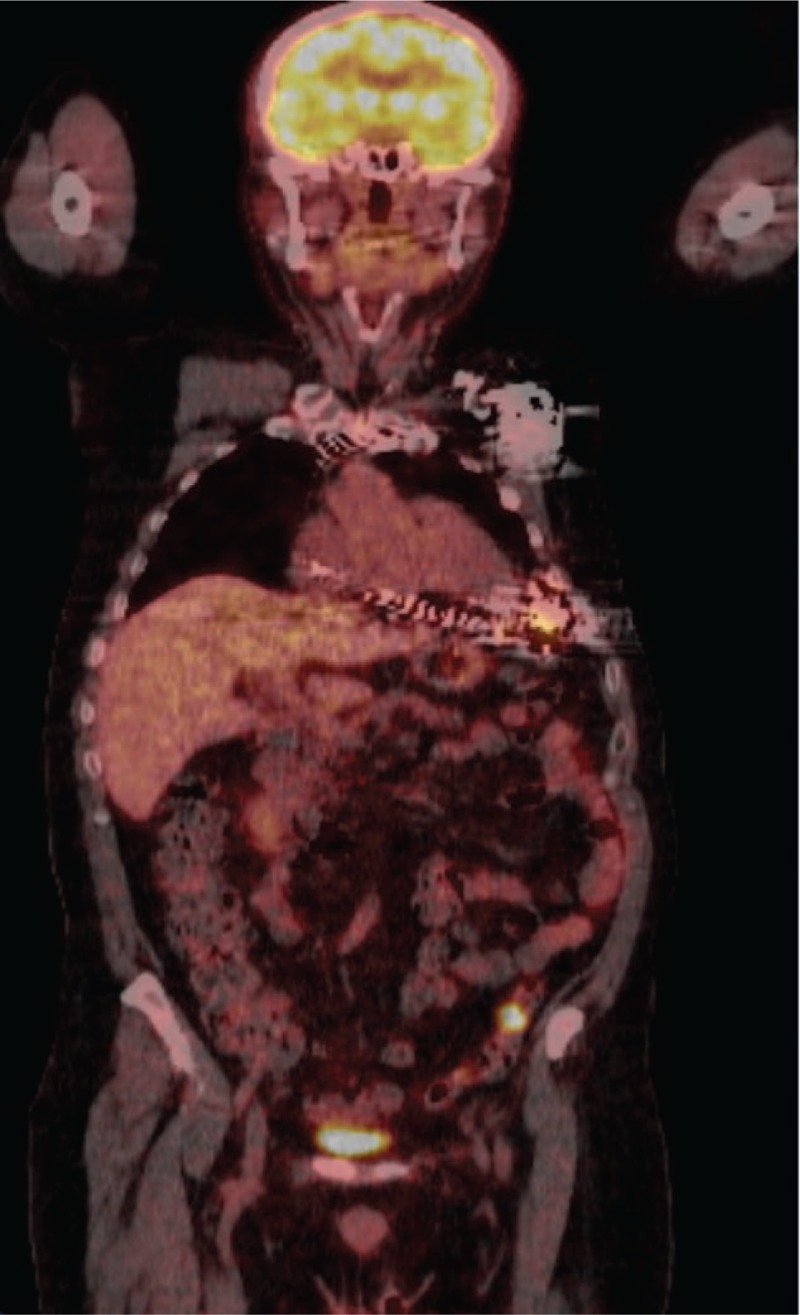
Positron emission tomography-computed tomography scan of patient presenting with *Sphingomonas paucimobilis* cardiac implantable electronic device infection.
